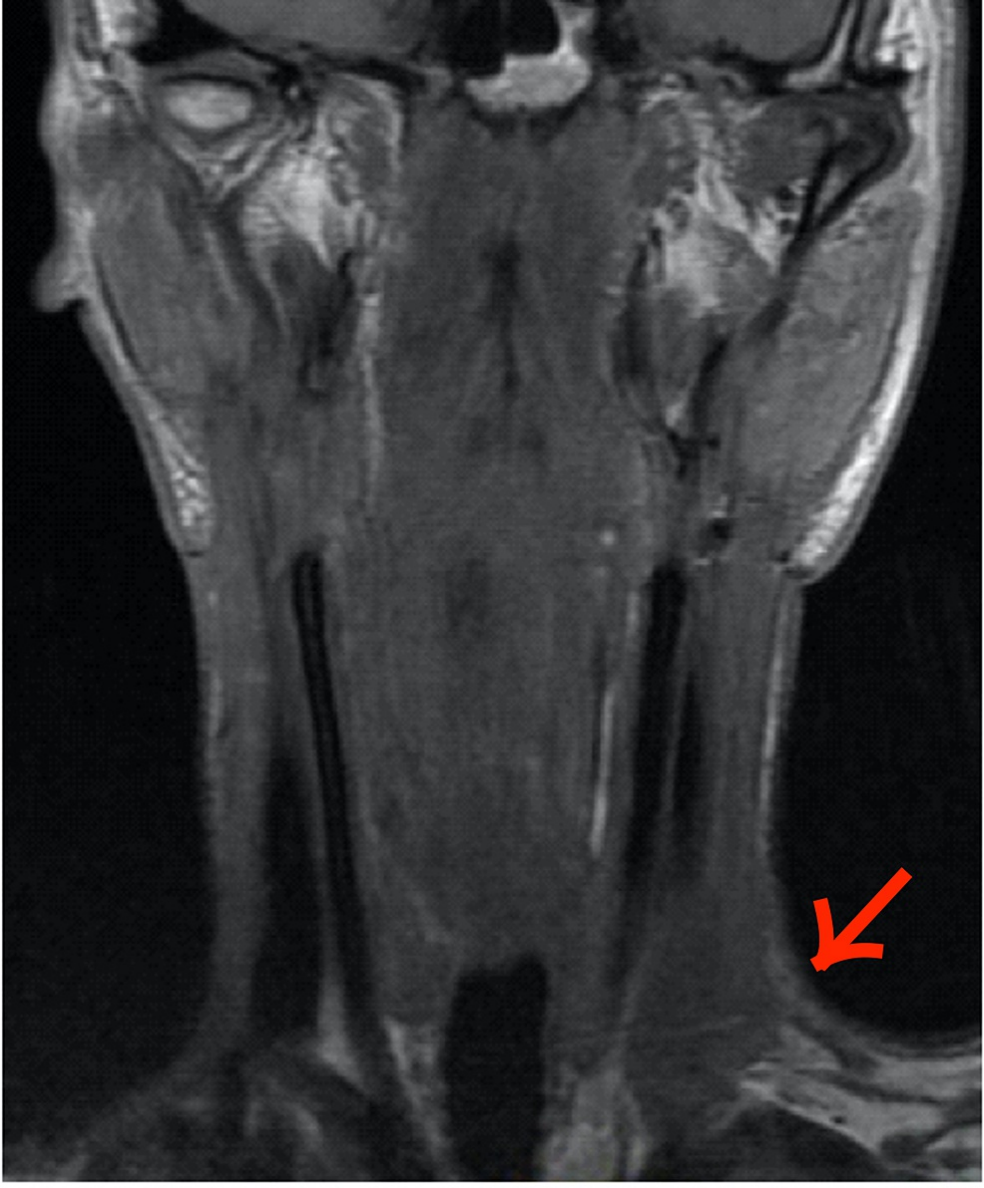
Arrow points to the chyloma on coronal T1-weighted, non-enhanced MRI of the neck. Together with Figure 1, this coronal view of the chyloma shows its location in left level IV of the neck where the thoracic duct is expected to be encountered during neck dissection. The chyloma appeared as a circumscribed hypointense lesion on T1.
